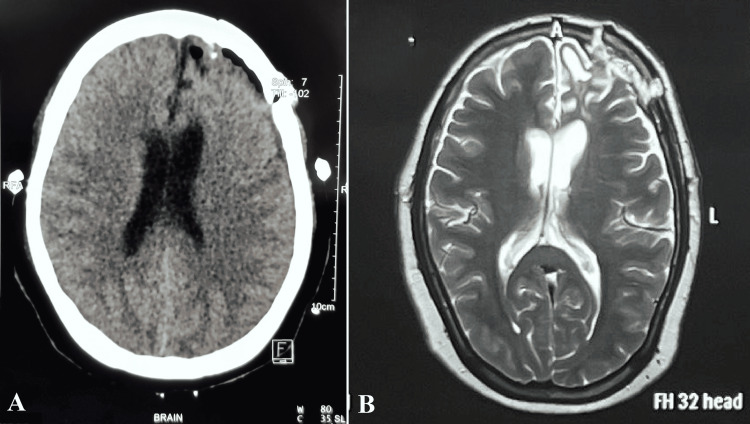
Postoperative axial (A) and sagittal (B) brain MRI images showing complete removal of the left frontal sinus osteoma and resolution of the pneumocephalus MRI: magnetic resonance imaging
